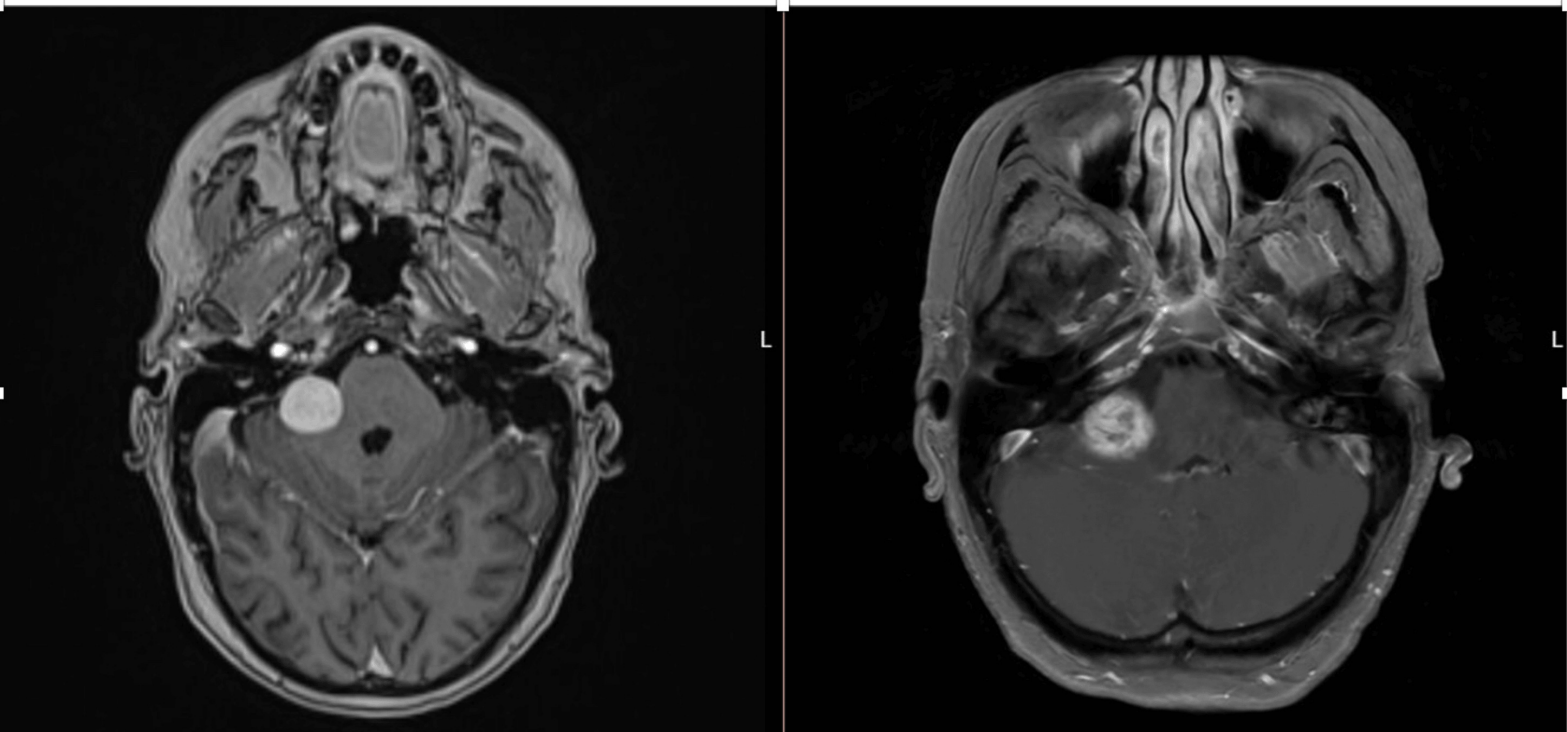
Axial T1-weighted MRI. Before radiotherapy (left), and 13 months after radiotherapy (right).
